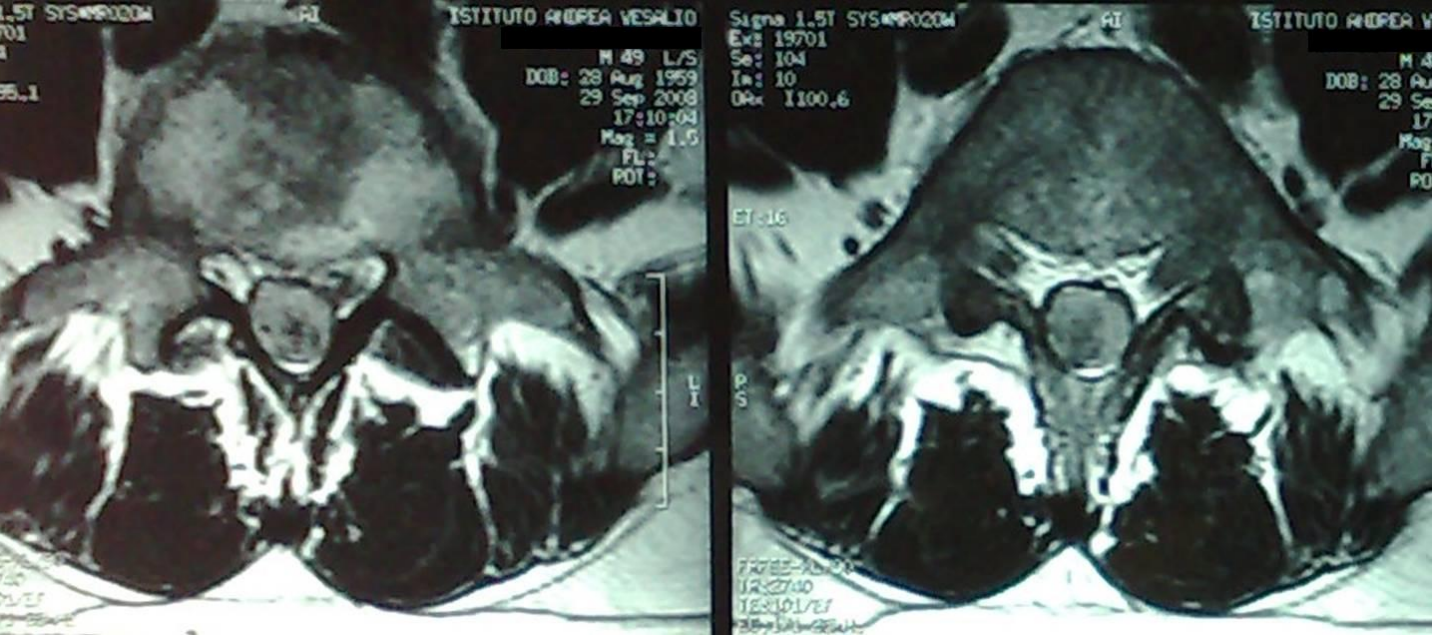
Preoperative MRI. Axial T1 weighted images with Gd-DTPA showed an enhancing well defined lesion at L4-L5 level
